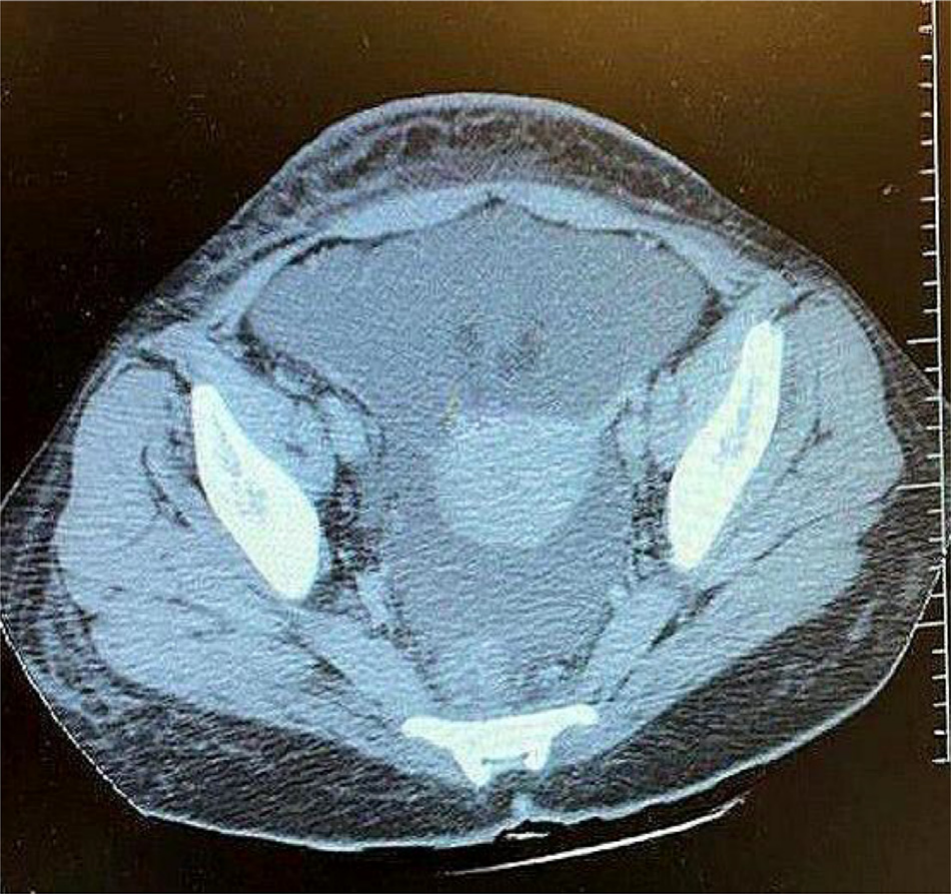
Pelvic CT scan: No significant pelvic mass or lymphadenopathy. Engorged pelvic veins, possibly due to venous congestion.
